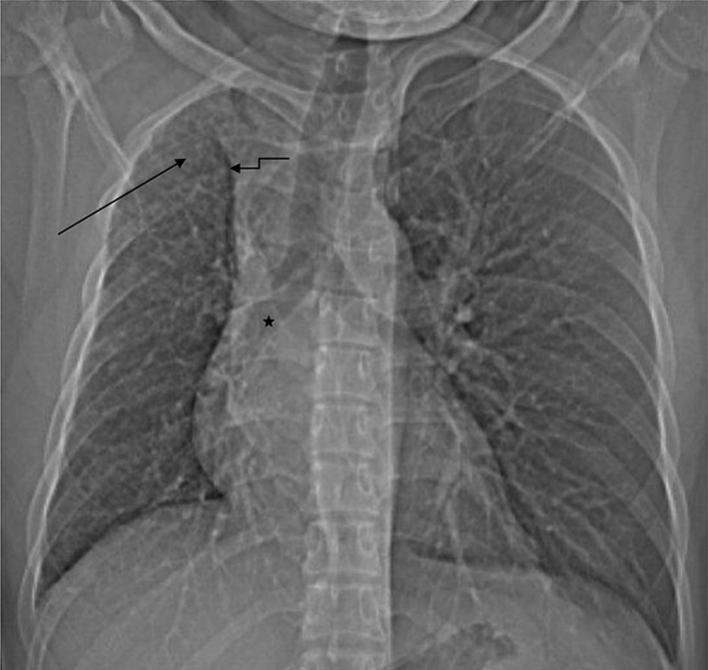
Chest radiograph shows small volume of the right lung, mild elevation of right hemidiaphragm, absence of the mediastinal portion of the right PA (
